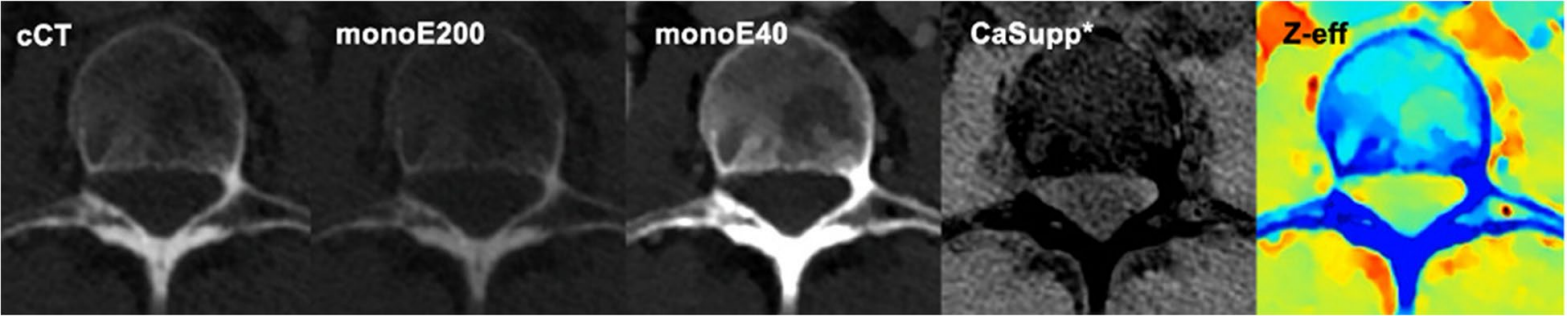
Conventional CT and dual-layer CT reconstructions of a 74-year-old female with breast cancer showing a vertebral osteolytic metastasis left posteriorly in the vertebral body of L3. It was best visible on monoE40, showed comparable visibility on Z-effective, reduced visibility on monoE200, and was not delineated on the Calcium suppression reconstruction. *Abbreviations: cCT, conventional CT; monoE200, monoenergetic 200 keV; monoE40, monoenergetic 40 keV; CaSupp, Calcium suppression; Z-eff, Z-effective. *Calcium suppression index of 55*
